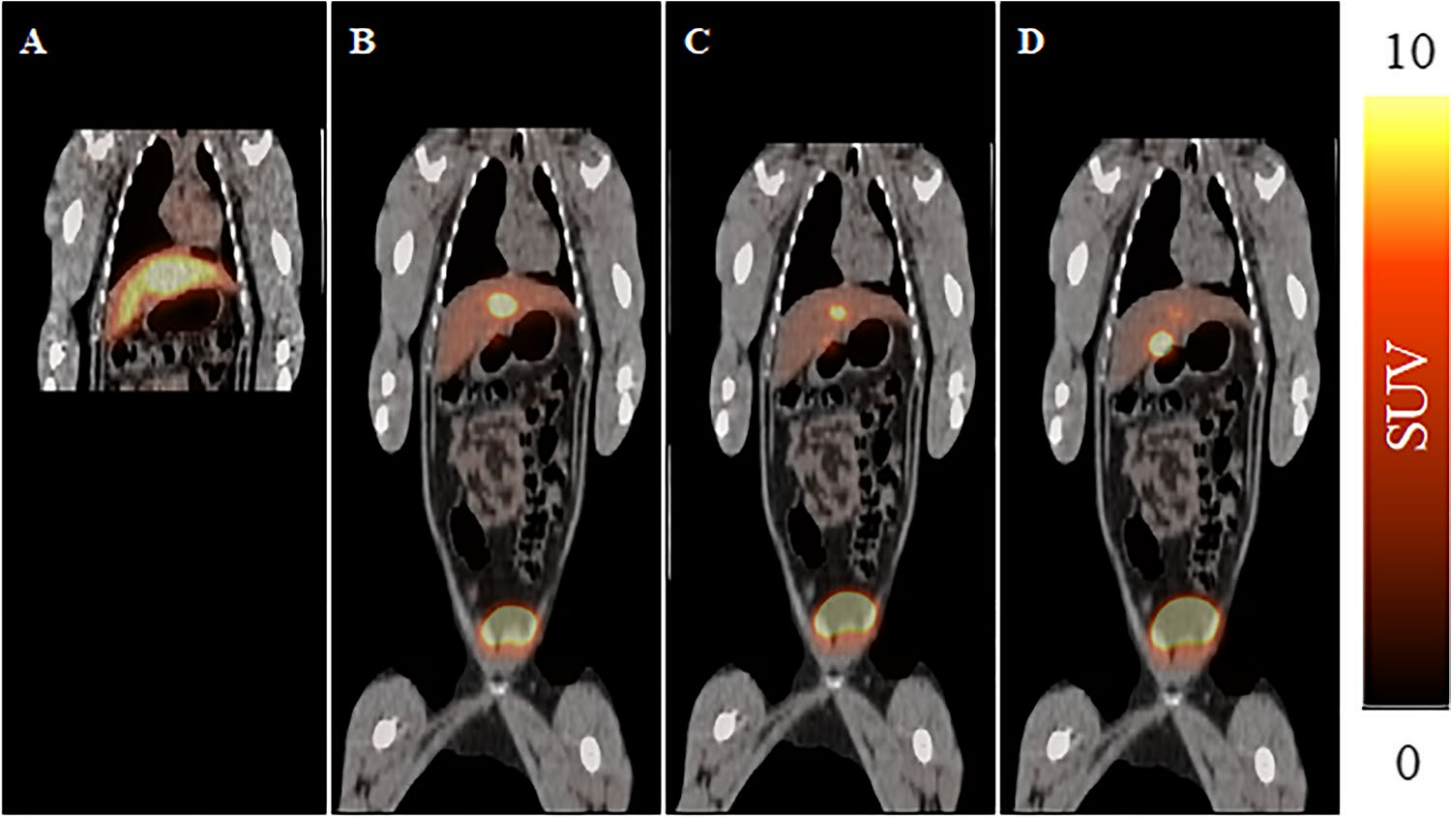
PET/CT coronal slice images following injection of [^18^F]FEtQ to a NHP. Images represent the summation of a 0–15-min, b 15–25-min, c 25–35-min, and d 35–45-min time frames.
